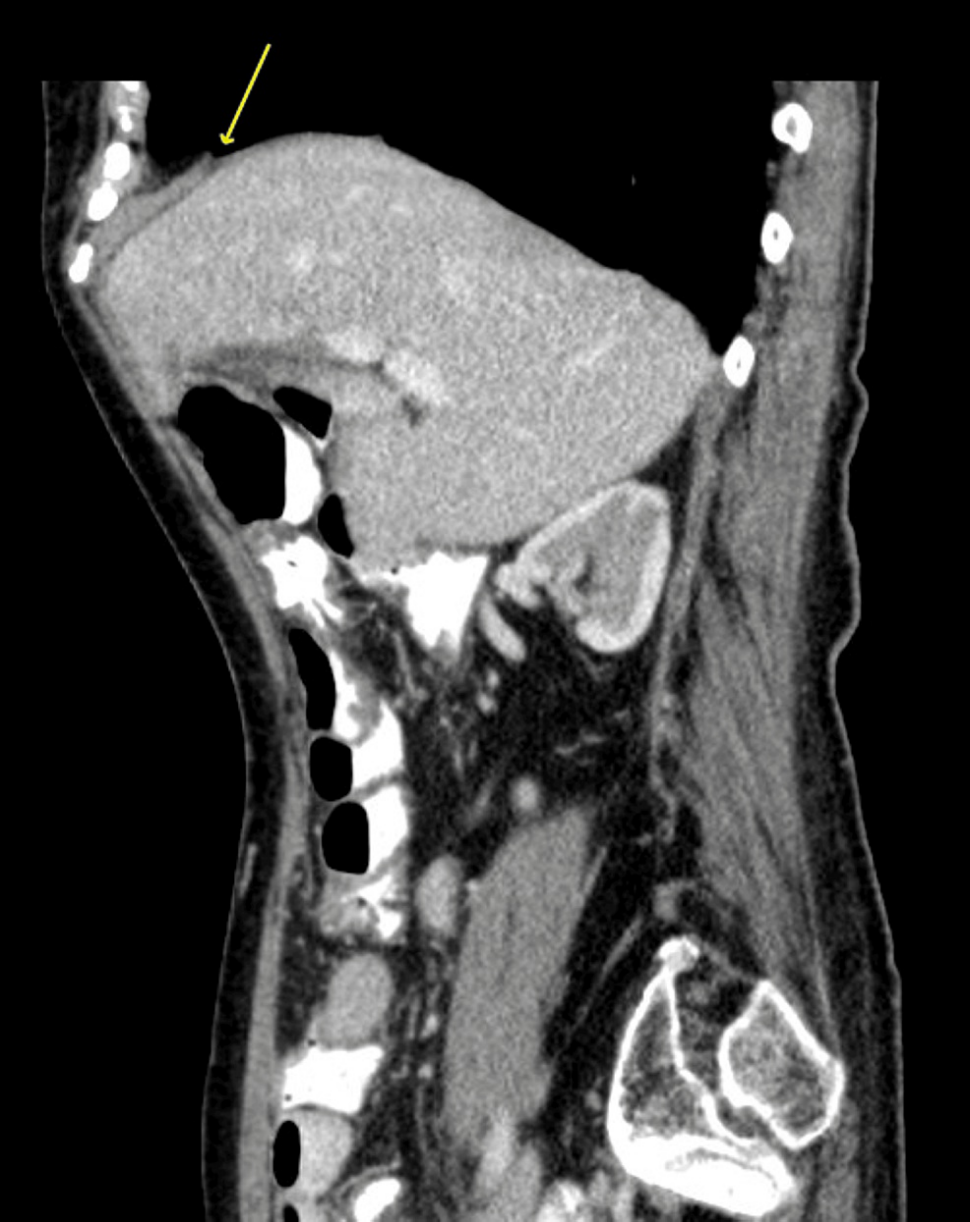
CT abdomen and pelvis from two years prior to presentation showing what appears to be a small diaphragmatic defect just anterior to the liver dome (see arrow).
